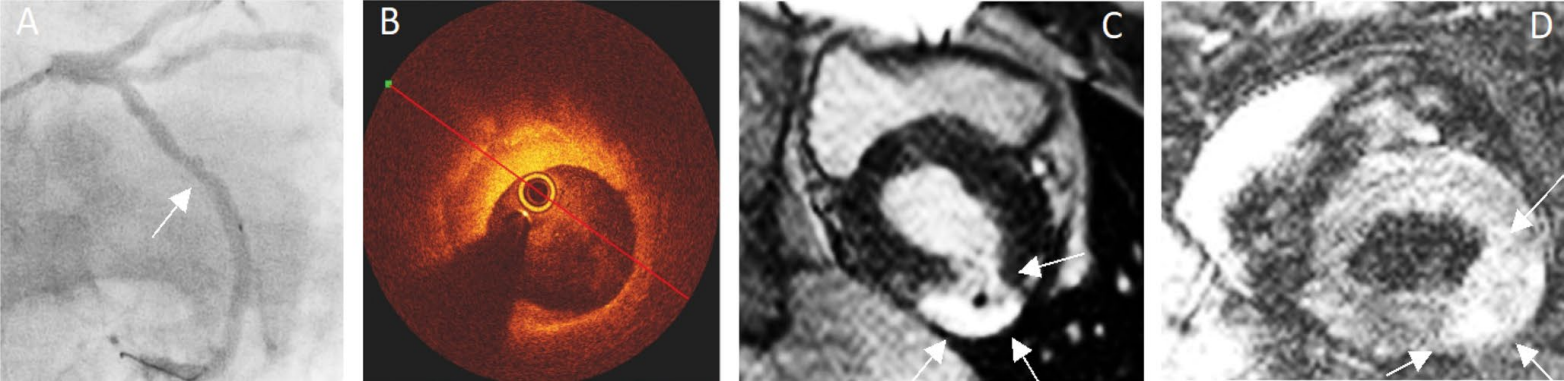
Organizing thrombus as the culprit in NSTEMI. Angiography of proximal LCX including OCT showing a 40% stenosis (A) and corresponding cross-sectional OCT (B) image shows an organizing thrombus due to a darker gradient towards the underlying luminal surface. Complementary CMR images (C; LGE image and D; T2-weighted image) of a single mid-ventricular short-axis slice: A large hyperenhanced area laterally (C, arrows) with a small black core indicating a large infarction with microvascular obstruction. The corresponding T2-weighted image (D) shows a hyperenhanced area with oedema laterally indicating acute infarction
